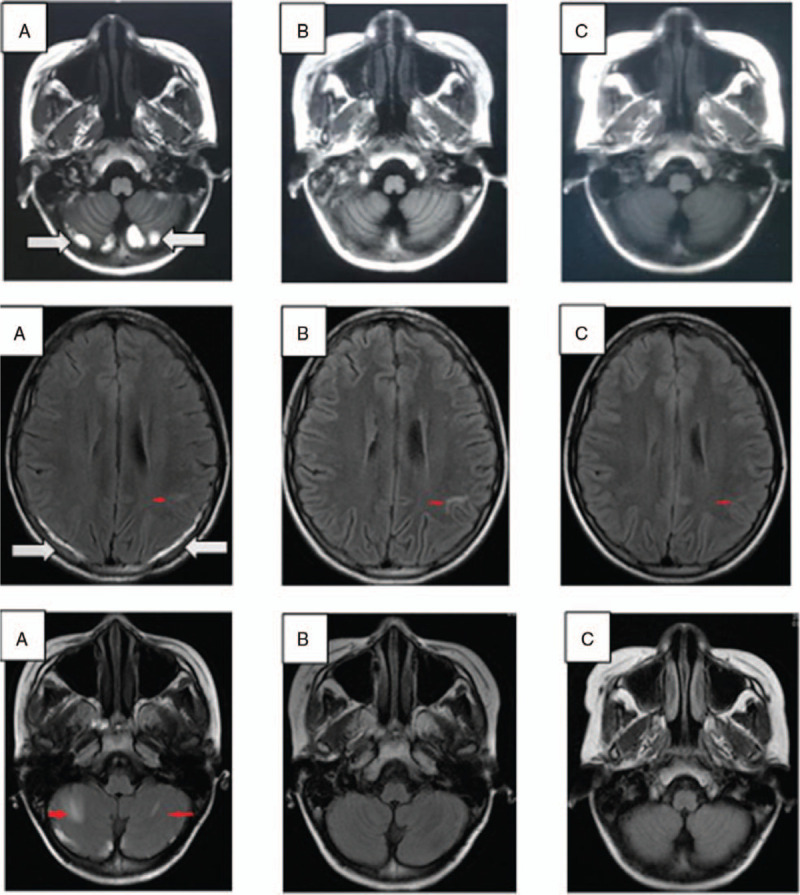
Magnetic resonance imaging (MRI) of the brain at different times. (A) Upon presentation, MRI showed a subacute subdural hemorrhage (white arrow) and multiple demyelinating lesions (red arrow) in bilateral cerebral hemispheres and cerebellum. (B) One month later and (C) 4 months later, MRI showed that the subdural hemorrhage had been absorbed completely, and multiple demyelinating lesions became smaller or disappeared in the cerebellum.
